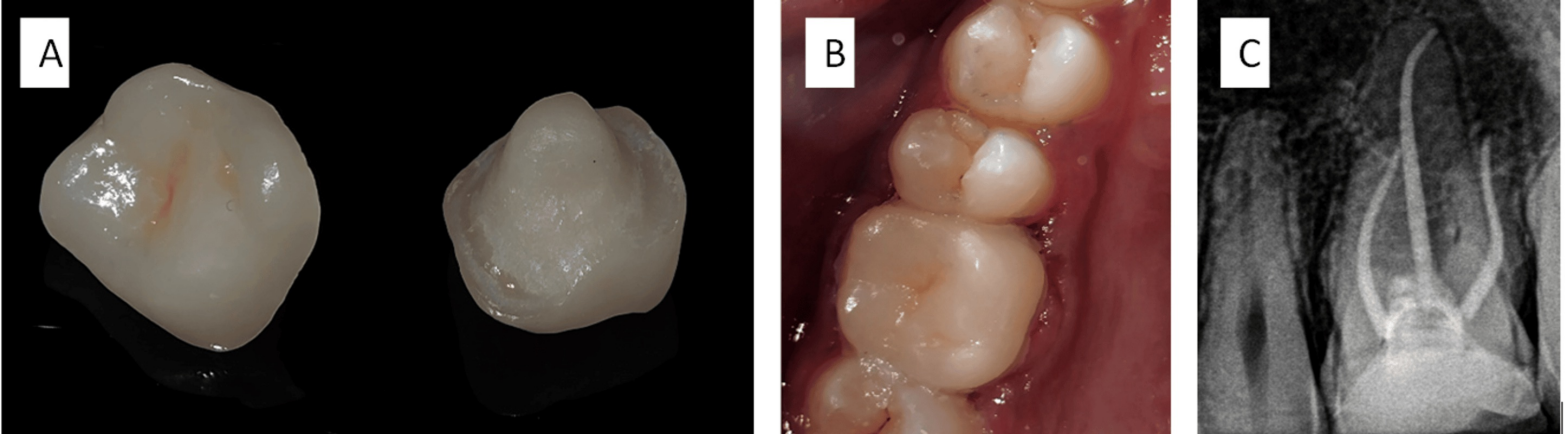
(A) Fabricated endocrown. (B) Clinical photograph showing the cemented endocrown with tooth number 16. (C) Radiograph following the cementation of the endocrown with tooth number 16.
